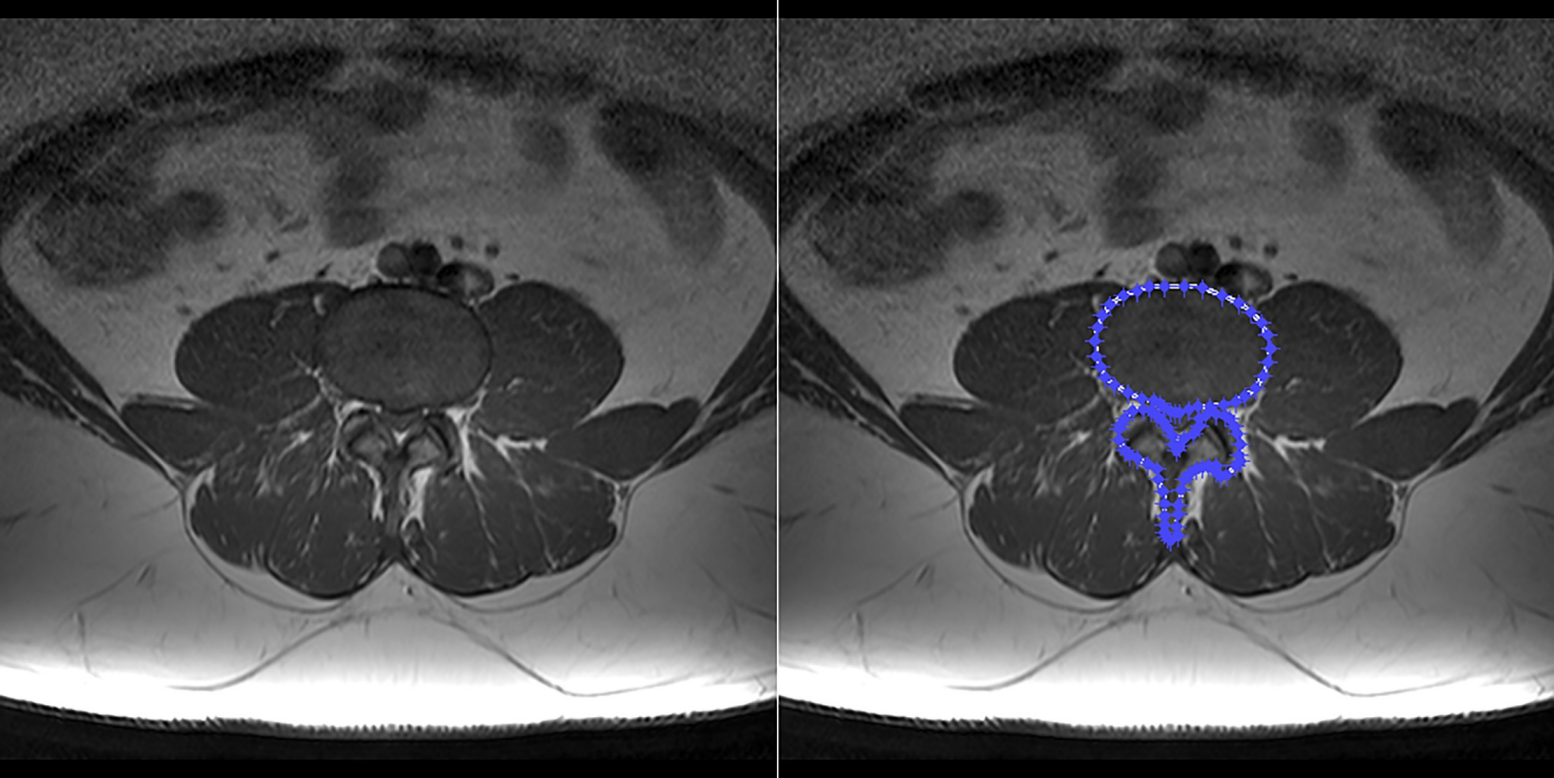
MR image and segmentation. An MR image is shown on the left and the same image is on the right with the vertebral bone outlined.
